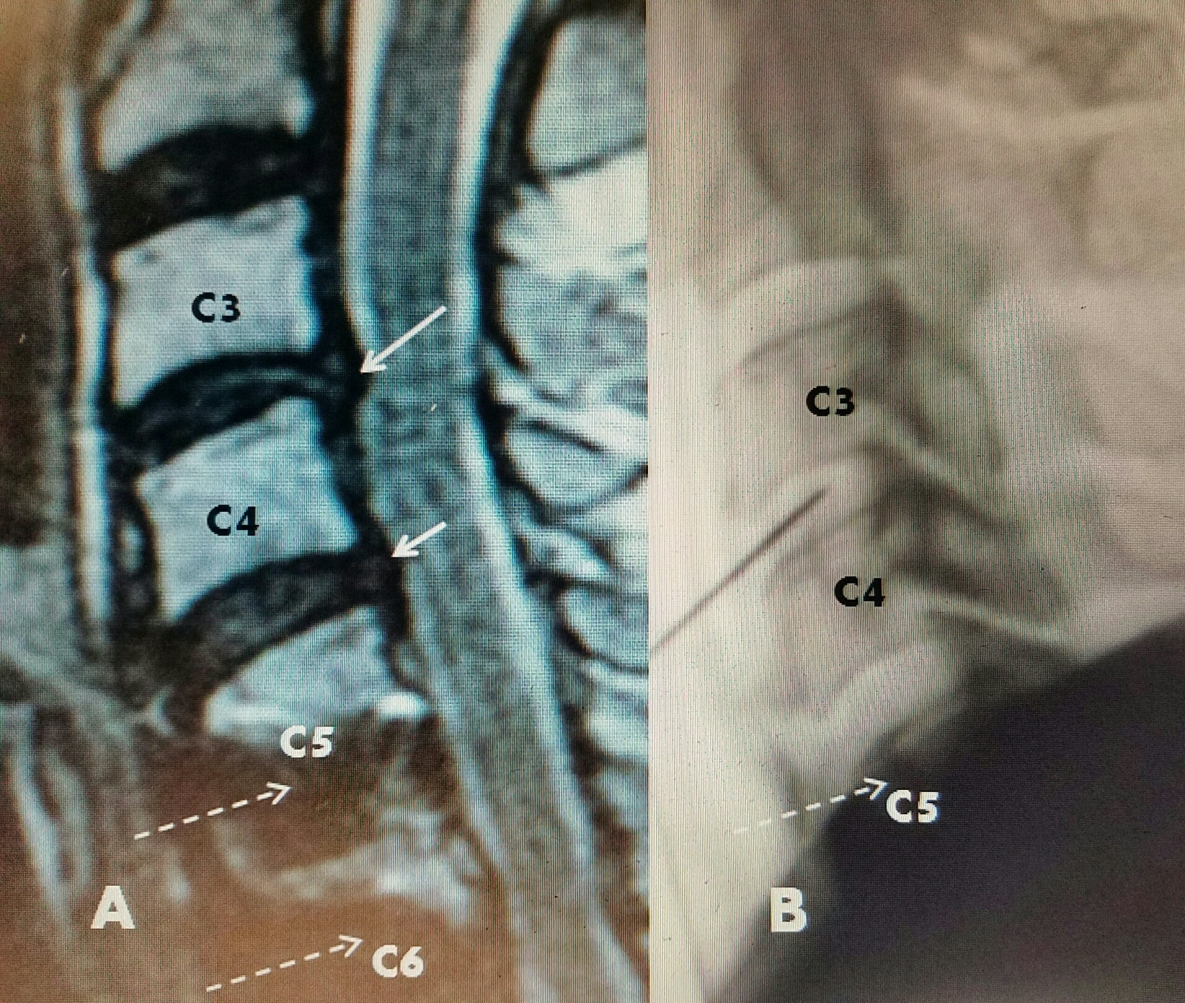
Patient #2: Previous C5-C7 ACDF and plating, new C3-4 disc A: T2 sagittal MRI: 2-3 mm C3-4 disc herniation (long white arrow) and smaller C4-5 protrusion (short white arrow). C5 and C6 anterior screws below (dotted white arrows). B: Intraoperative picture of discectomy probe within C3-4 disc space. The probe can move within the disc space making contact with both superior and inferior endplates. Debridement can be performed with endplate contact. ACDF: Anterior cervical discectomy and fusion; MRI: Magnetic resonance imaging.
